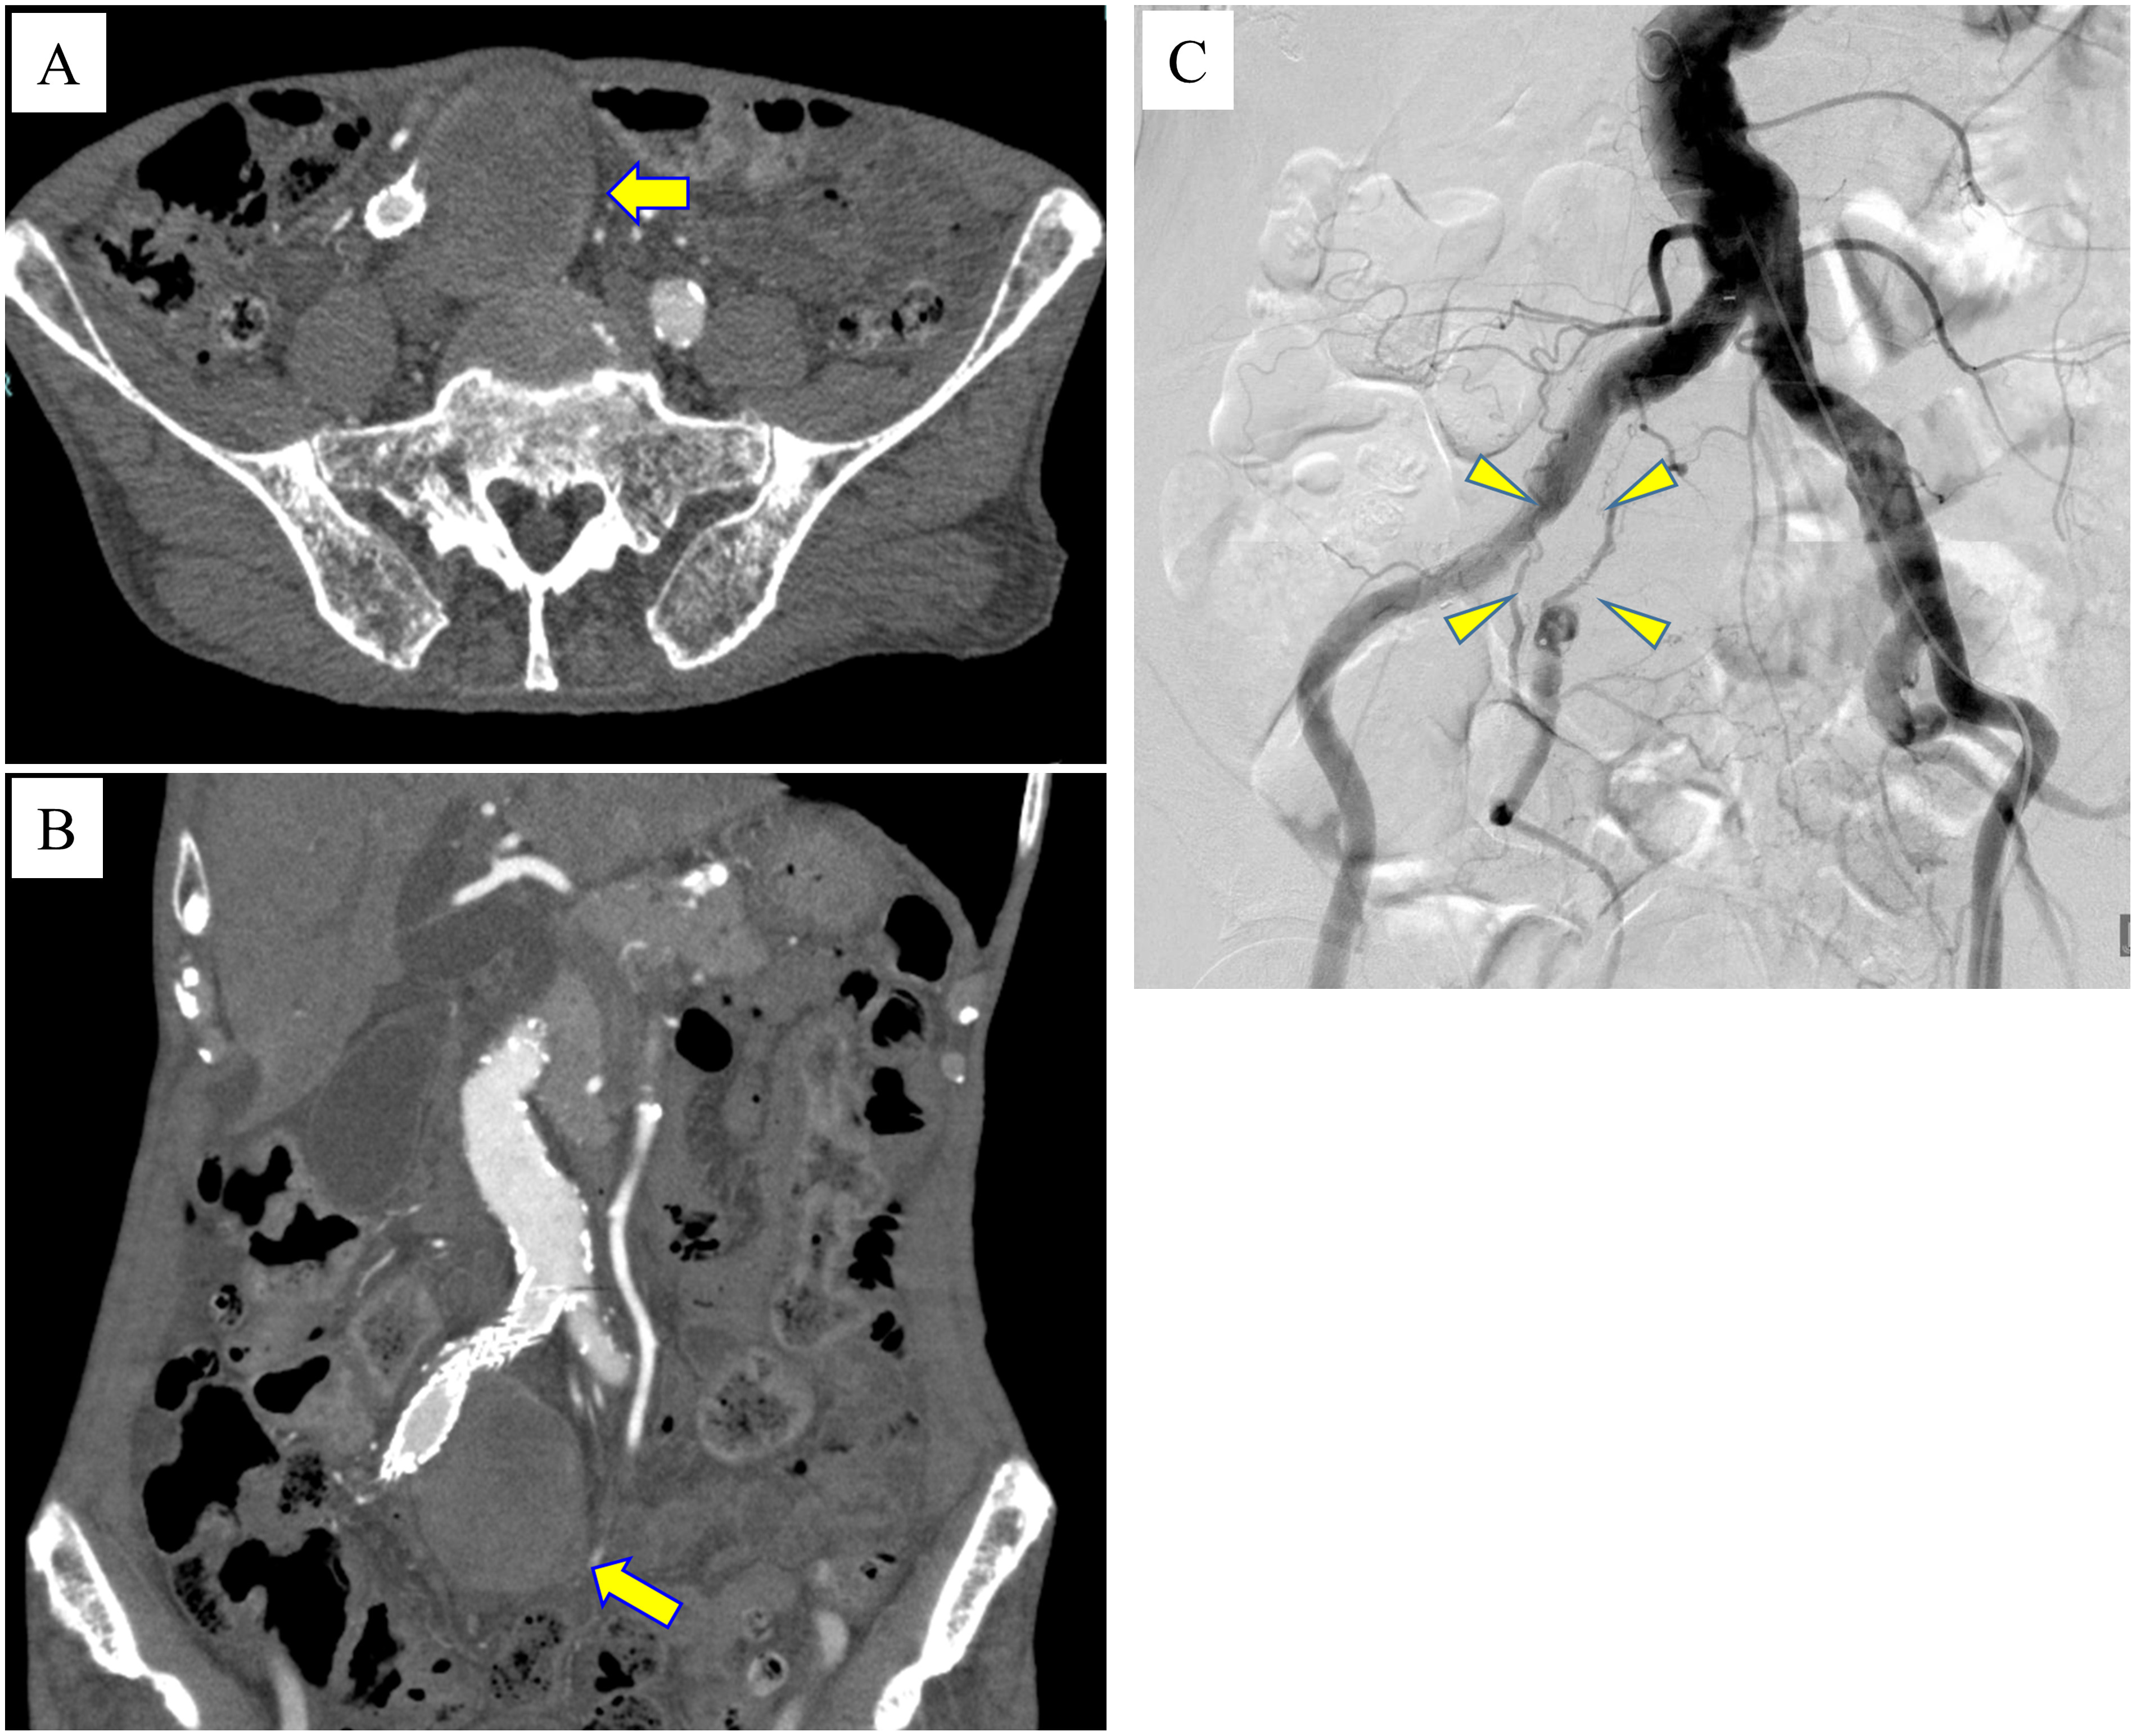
Fig. 2 (A), (B) Computed tomography 2 years after endovascular treatment showing a 50 mm right internal iliac artery aneurysm with no obvious endoleak. (C) Angiography showed no findings of endoleak 2 years after endovascular repair.
